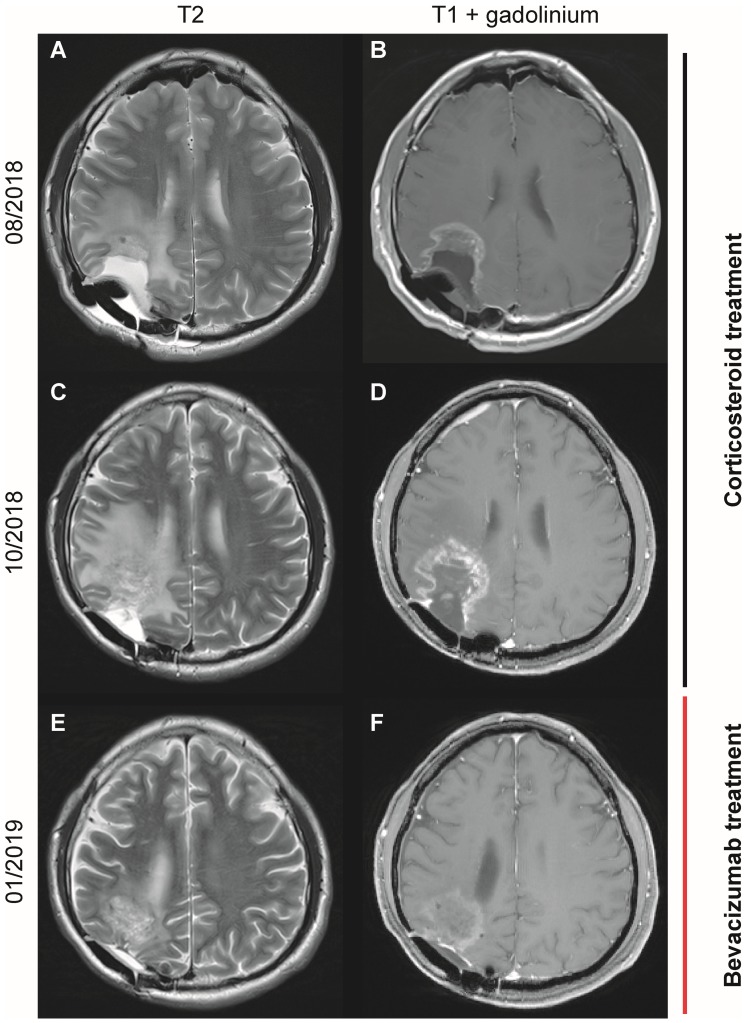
Brain edema and disruption of the blood–brain barrier in an index patient with cerebral radiation necrosis. MRI scans of a 46-year-old patient with glioblastoma of the right parietal lobe (A,C,E): T2 weighted images (WI); (B,D,F): T1 (WI) after intravenous gadolinium). Re-radiation was applied 10 months before the first MRI. (A,B) MRI shows a non-solid, necrotizing lesion with rim enhancement adjacent to the resection defect with surrounding edema. Due to worsening headache and increased rate of epileptic seizures, dexamethasone was started one month later. (C,D) the clinical symptoms had declined, yet the MRI shows an increasing extent of the rim-enhancing lesion and of the edema, so therapy with bevacizumab was started one month later. (E,F) the first follow-up MRI after two infusions of bevacizumab showed a significant reduction of contrast enhancement and edema. Treatment with dexamethasone could be stopped shortly after, but serum analysis revealed an adrenal insufficiency.
